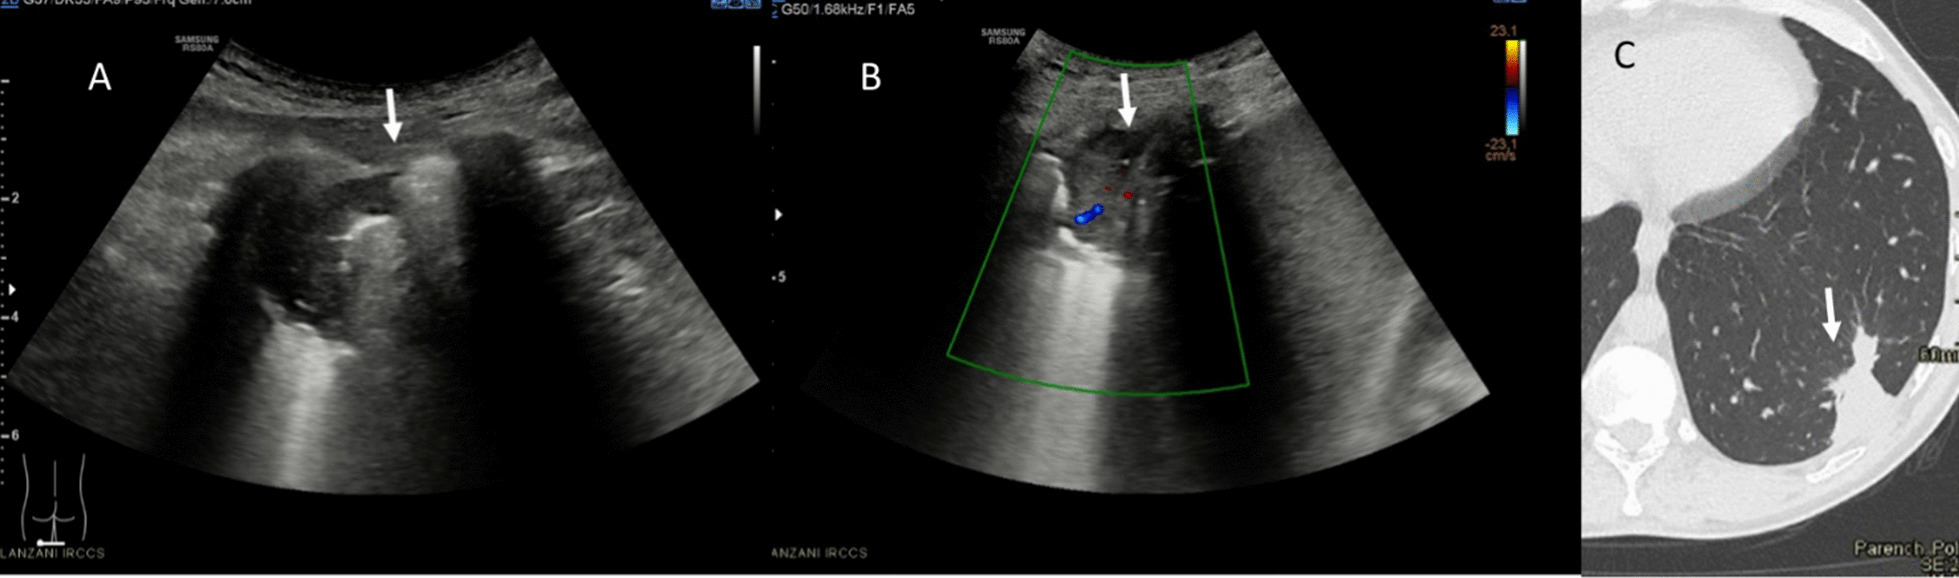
Delta patient: in A (US assessment) and B (Color-Doppler assessment) consolidative pattern (arrows) in L6; Lung Score 24. On CT assessment (C), arrow shows consolidative pattern
